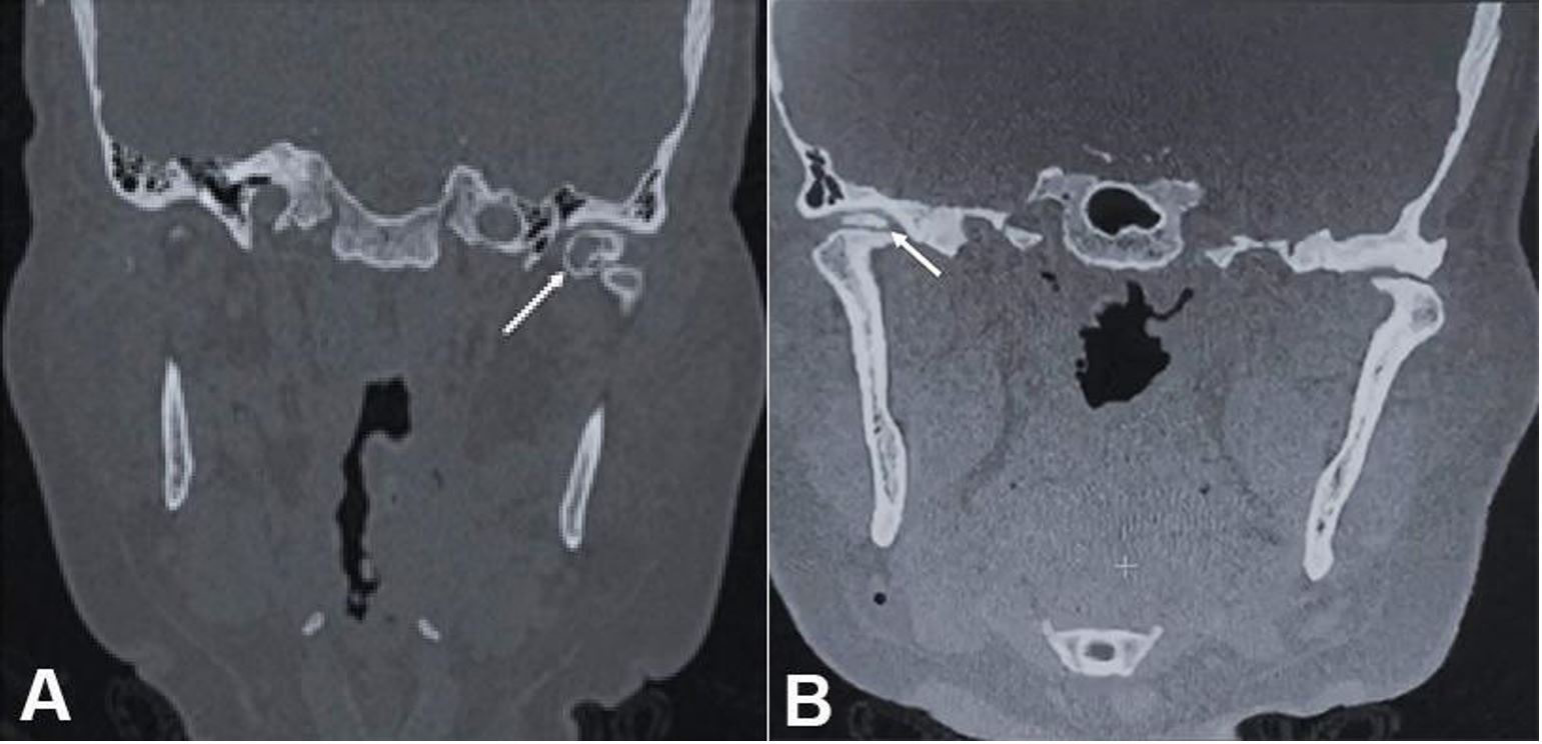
A – Preoperative computed tomography coronal section TMJ left side showing loose body (arrow); B – Preoperative computed tomography coronal section TMJ right side showing loose body (arrow).
